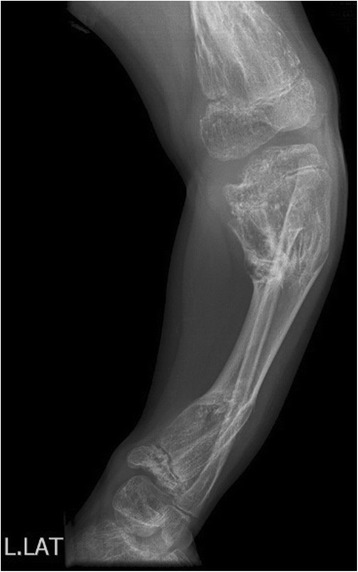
Lateral radiograph of left lower limb: bone deformity with multiple lytic lesions in the upper and lower metaphyses of tibia and fibula as well as distal end of femur
